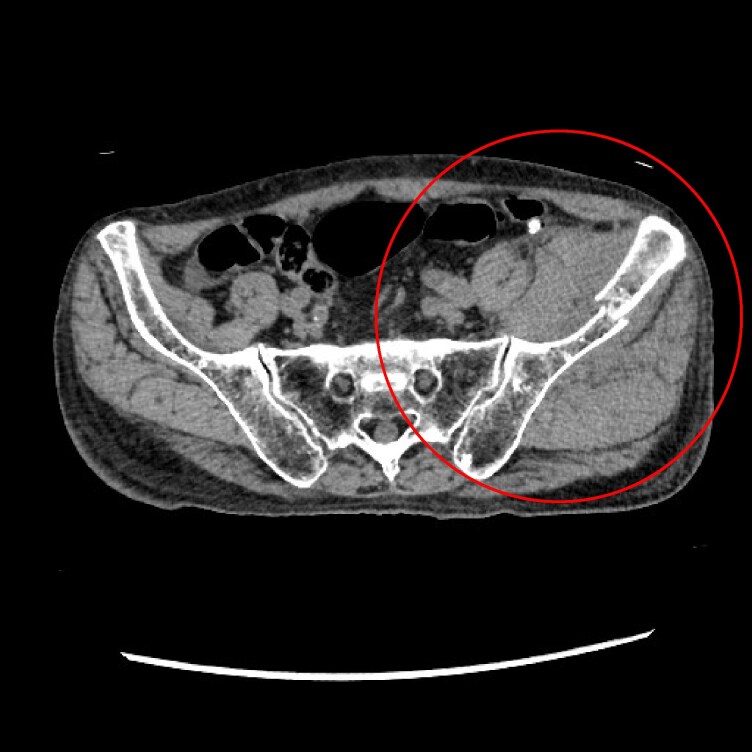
Contrast-enhanced computed tomography image showing a left iliac bone fracture with an adjacent expanding haematoma (highlited area).
